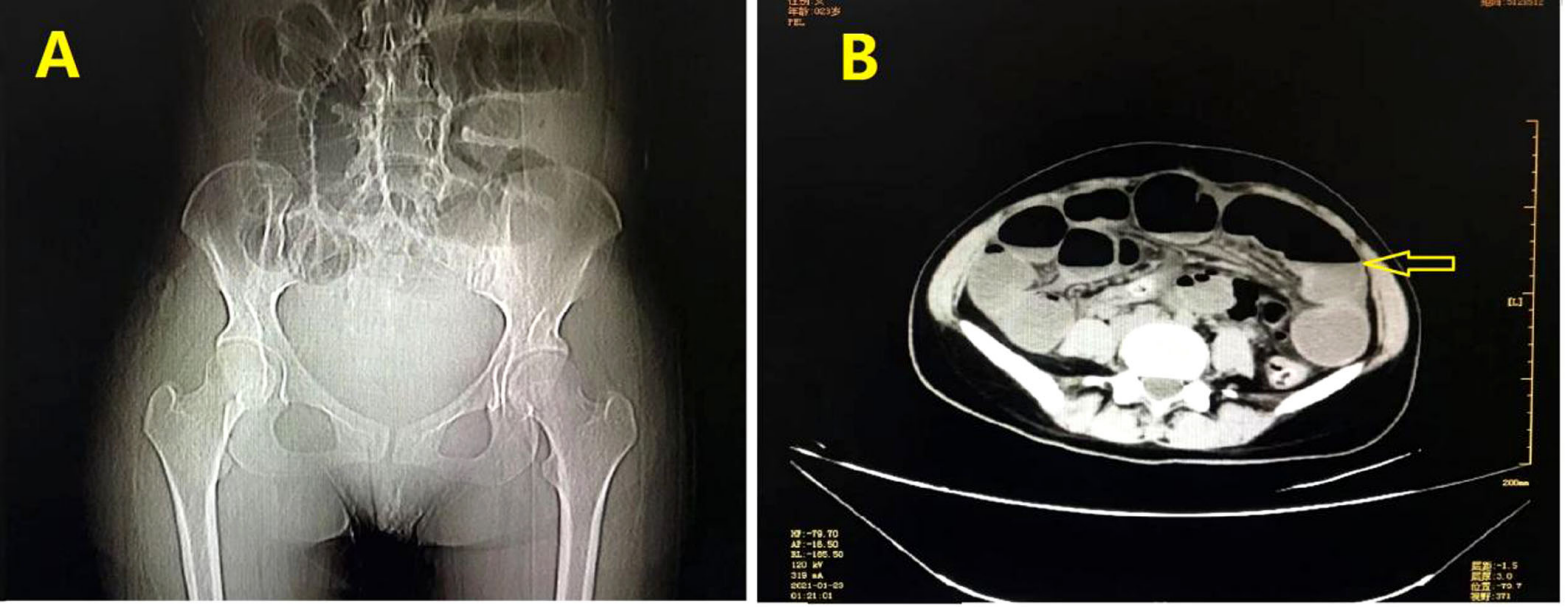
Imaging examination: (A) Digital radiology and CT scanning showed gas and fluid accumulation in the small intestine and indicated intestinal obstruction. (B) Liquid, air and high intestinal tension were visible (lesion was marked with yellow arrow).
